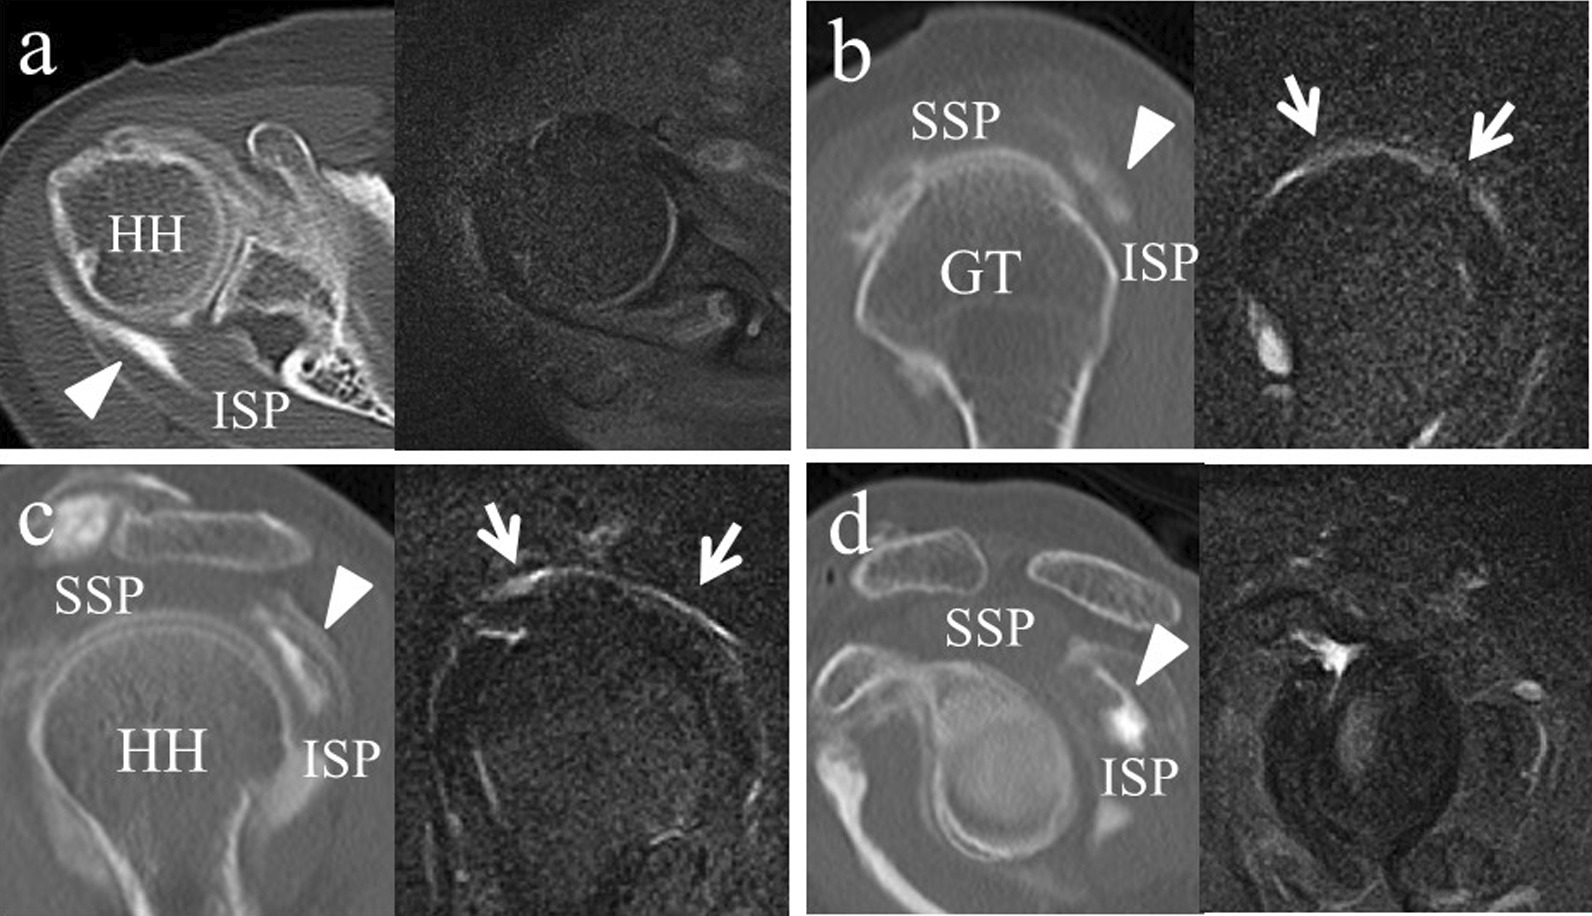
The tear is clearly visualized by Computed tomography bursography, although on fat-suppressed T2-weighted Magnetic resonance imaging, there appears to be increased signaling in the bursa, suggesting bursitis. On Computed tomography bursography, (a) axial image shows longitudinal pooling of contrast medium in the infraspinatus (arrowhead). b On Computed tomography bursography, the oblique sagittal image shows that the tear communicates with the subacromial bursa near the insertion of the infraspinatus tendon (arrowhead) and (c, d) within the infraspinatus tendon and muscle (arrowhead). Magnetic resonance imaging shows areas of increased signal in the bursa (arrows). *HH* head of the humerus, *GT* greater tubercle, *SSP* supraspinatus, *ISP* infraspinatus
